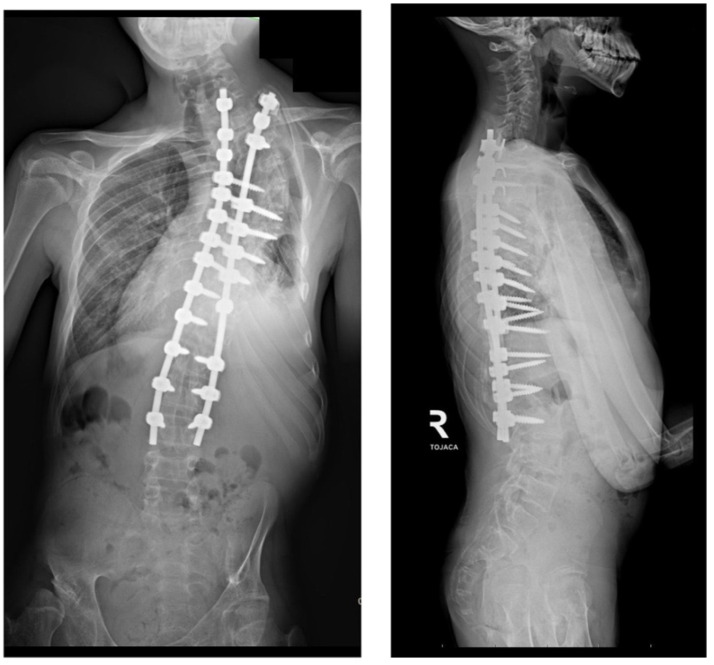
Radiological standing X-rays AP and LAT of the patient with Costello syndrome at 2 years of follow-up after undergoing conversion from MCGR to posterior spinal fusion.
